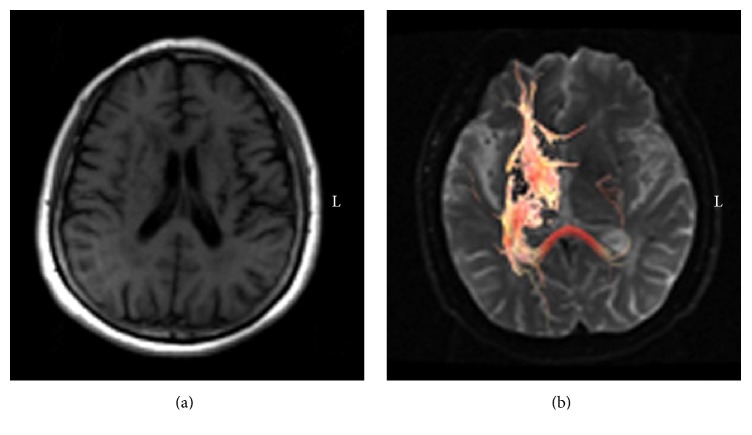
Follow-up radiographs of Case  4 in the chronic phase. (a) T1-weighted images show the encephalomalacic foci in the posterior limb of the left internal capsule; (b) an additional diffusion tensor tractography shows the complete interruption of the corticospinal tract in the posterior limb of left internal capsule.
